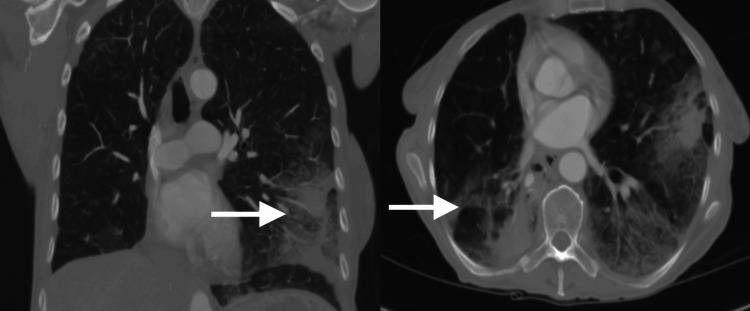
Coronal (left) and axial (right) chest CT views of the infected patient. White arrows depict diffuse bilateral infiltrates with ground-glass opacity and bibasilar pleural effusions. CT: computed tomography
